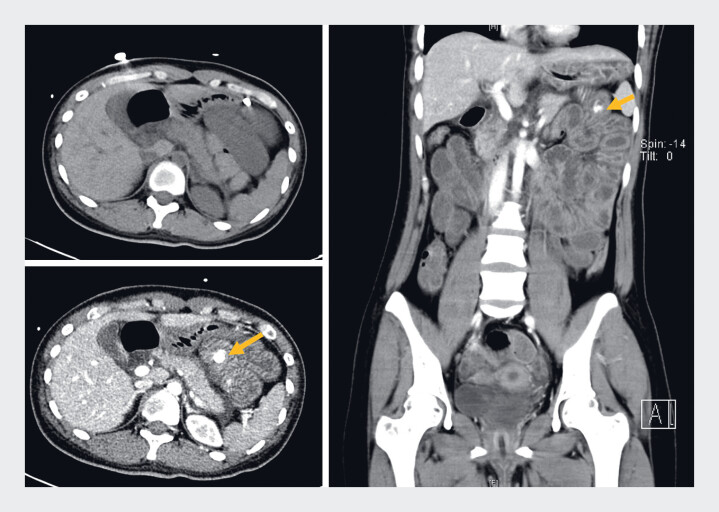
Computed tomographic angiography revealed a dense shadow (arrows) in the bowel lumen in the left upper abdomen.
